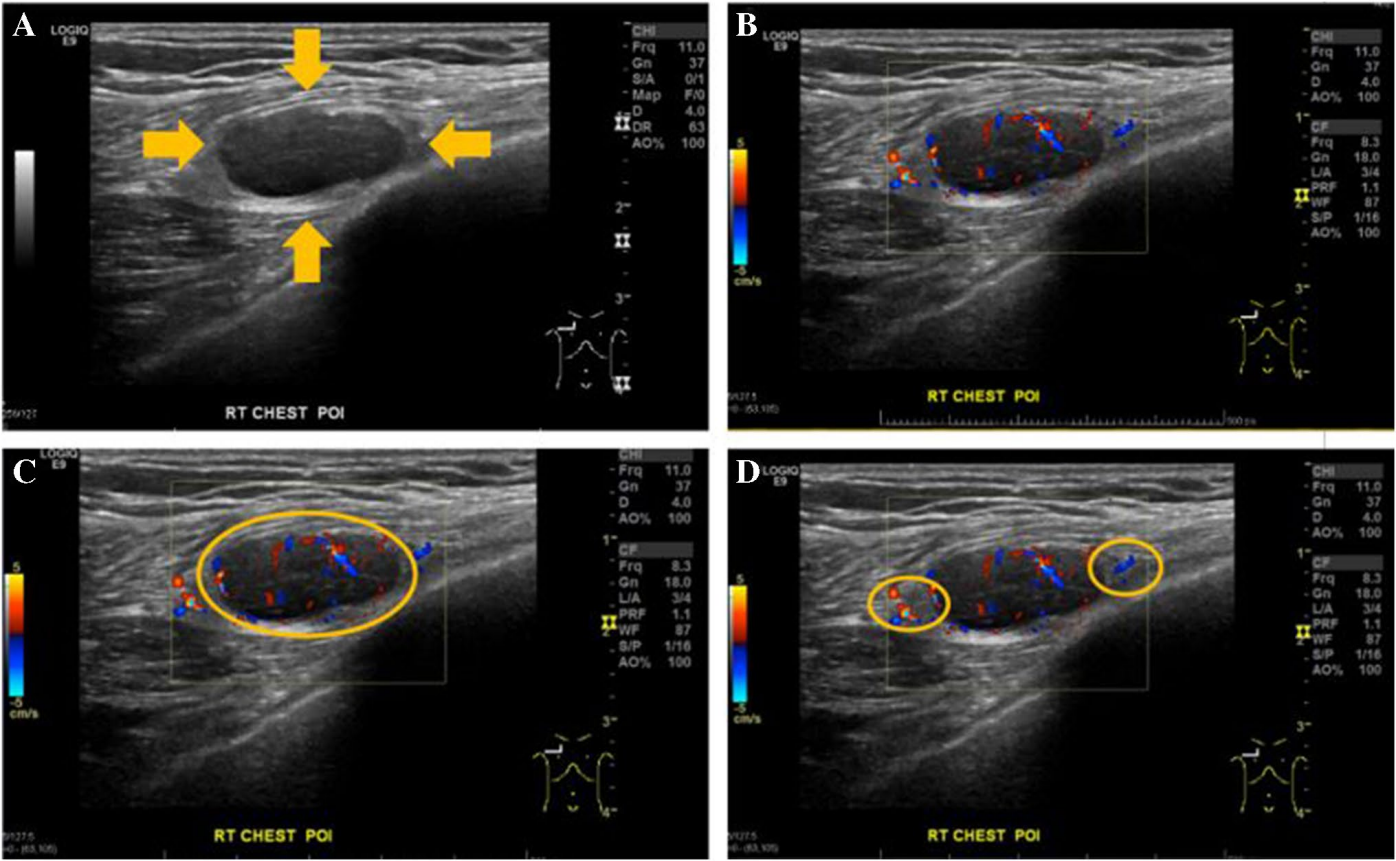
Ultrasound of chest wall mass. A Obtained due to pain in the area despite the only vague radiograph findings. Gray-scale ultrasound demonstrates a sharply demarcated, homogeneous, hypoechoic chest wall intramuscular mass without internal calcification (arrows). B–D Color Doppler imaging (B–C) demonstrates internal vascularity (large yellow circle) and possible peripheral feeding vascular pedicle (smaller yellow circles in D)
